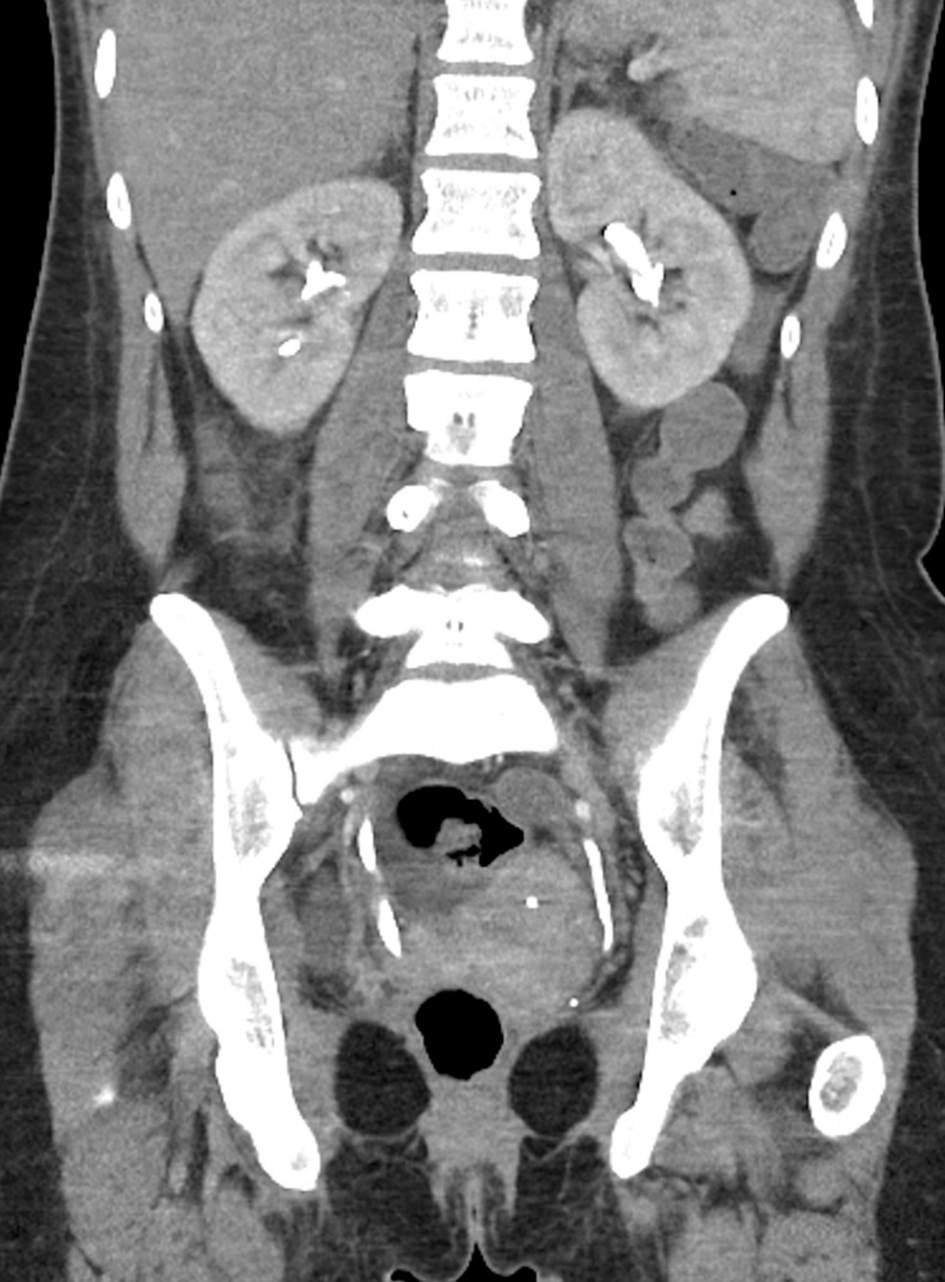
Coronal view of CT urogram showing no evidence of hydronephrosis on either side with normal contrast excretion
